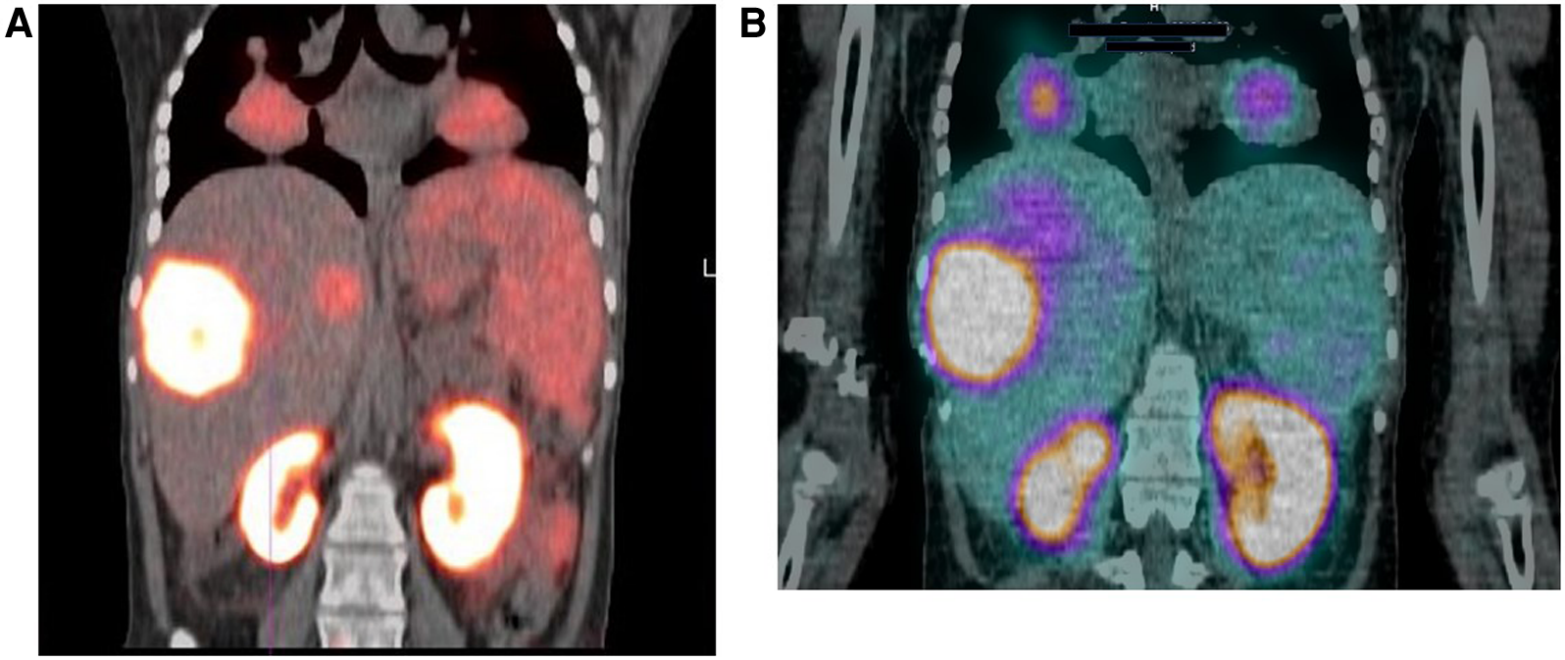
18-year-old female with a 2-year history of HCC. The patient had a hepatectomy and subsequent recurrence, which was not responding to conventional therapy. (A) Ga-68 PSMA scan demonstrating uptake in the liver and lung lesions. (B) Lu-177 PSMA post-therapy image, demonstrating congruent uptake in these lesions.
